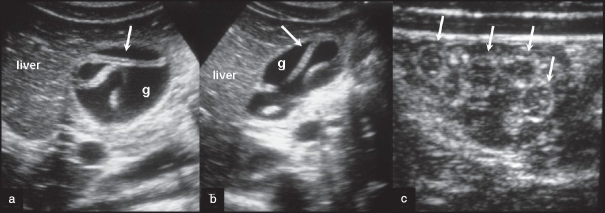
Ultrasonography of the right hypochondrium in transverse (a) and longitudinal (b) planes showing the gall bladder (g). Sonogram of one of the small bowel loops (c) shown with linear transducer
